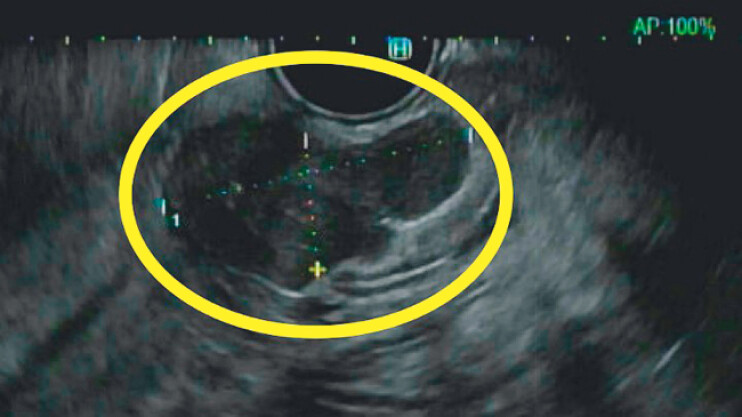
Endoscopic ultrasound (EUS) showing a 2.5-cm GIST.
